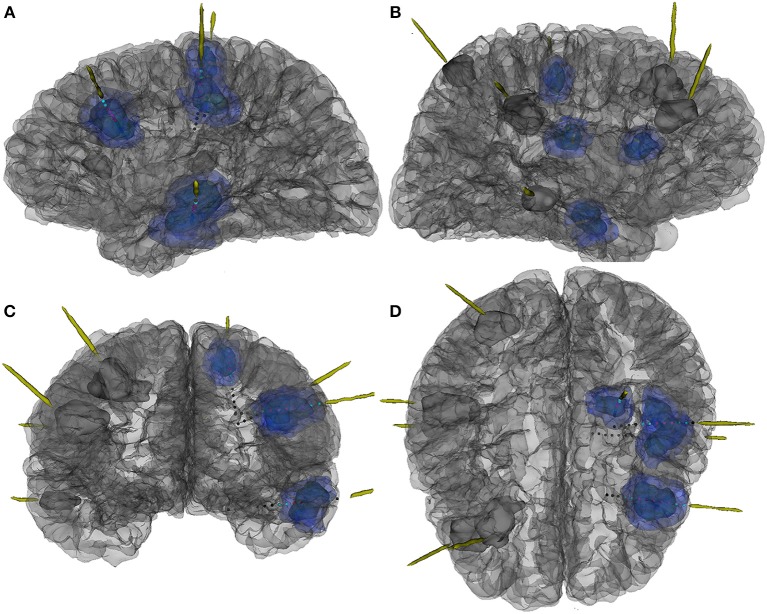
The 3D reconstruction of MR and CT fusion image to show location of electrodes and cortical tubers and their relationships in patient 7. (A) Left side view, (B) right side view, (C) front view, and (D) top view. Dark blue mass, the center part of tubers; light blue mass, the peripheral part of tubers; grey mass, the tuber without CCEP examinations; red dot, contacts in the center part of tubers; blue dot, contacts in the peripheral part of tubers; grey dot, contacts out of tubers.
